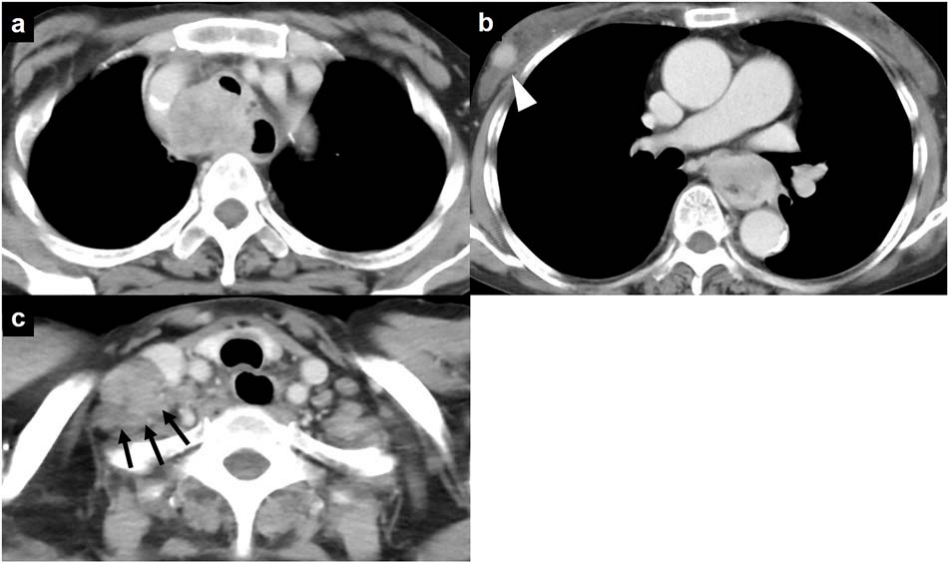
Chest computed tomography of a 59-year-old woman who was admitted to our hospital; (a) a mass is visible invading the trachea in the upper mediastinum; (b) an esophageal mass and a mass in the subcutaneous tissue of the right chest wall (arrowhead) are visible; (c) a mass in the right cervical region is also present (black arrows).
